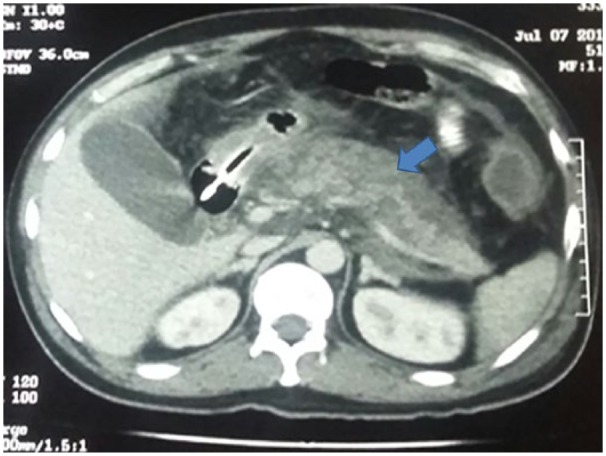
Abdominal computed tomography scan from the episode of acute pancreatitis 2 months ago showed necrotizing acute pancreatitis (blue arrow).
